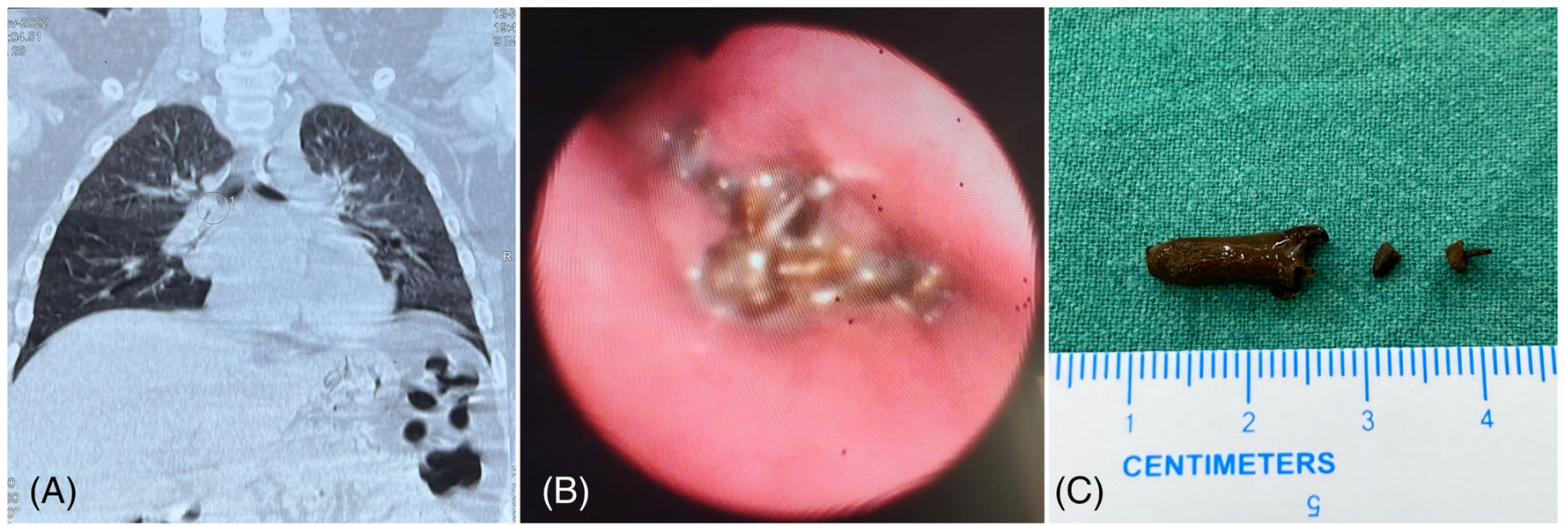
(A) Coronal plane CT showing obstruction in the right intermediate bronchus, (B) endobronchial view at the level of right main bronchus showing impacted clove with granulation tissue in the proximal right intermediate bronchus, and (C) retrieved clove
